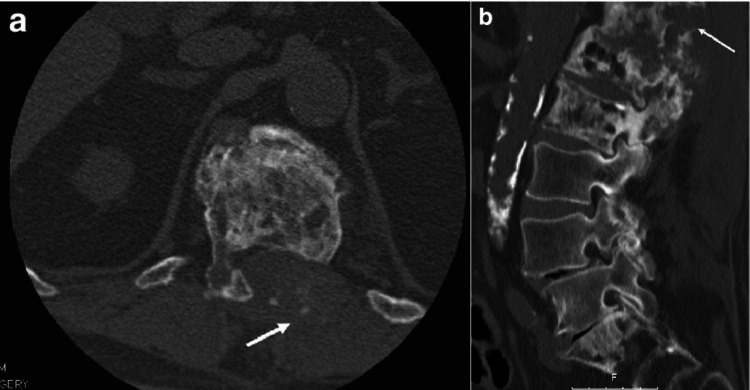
Computed tomography scan showing osteosarcoma in patient with history Paget's disease of bone (PDB) in the lumbar spine. Notice the extensive lytic pattern of destruction of the L1/2 vertebrae, which is pathologic for PDB. The arrows point toward an expanding mass that is the site of osteosarcoma secondary to PDB Reprinted from Sofka et al. [47], Copyright © 2006 by Sage Publications, with permission from Sage Publications.
